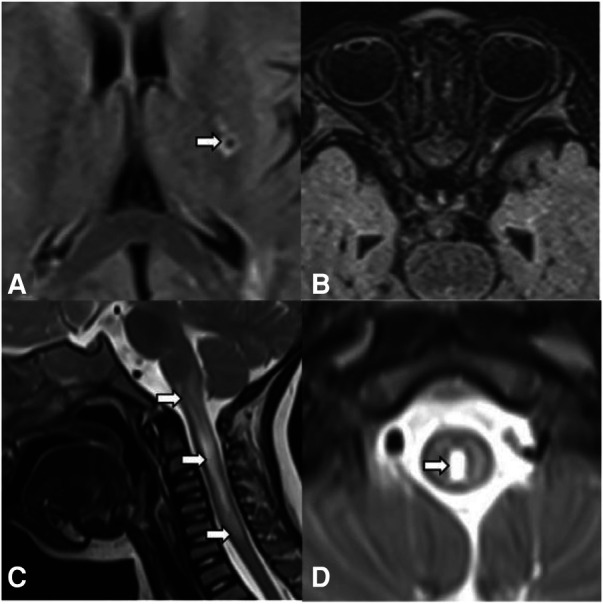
Brain MRI, spinal cord MRI, and optic MRI of the patient. Axial T2-weighted fluid-attenuated inversion recovery (FLAIR) MRI shows small encephalomalacia foci with gliosis in the left basal ganglia (A, arrows) and normal optic nerves (B). Sagittal (C) and axial (D) T2-weighted MRI of the spinal cord demonstrates longitudinally extensive transverse myelitis (LETM) lesion involving medulla to C6 leve (arrows).
